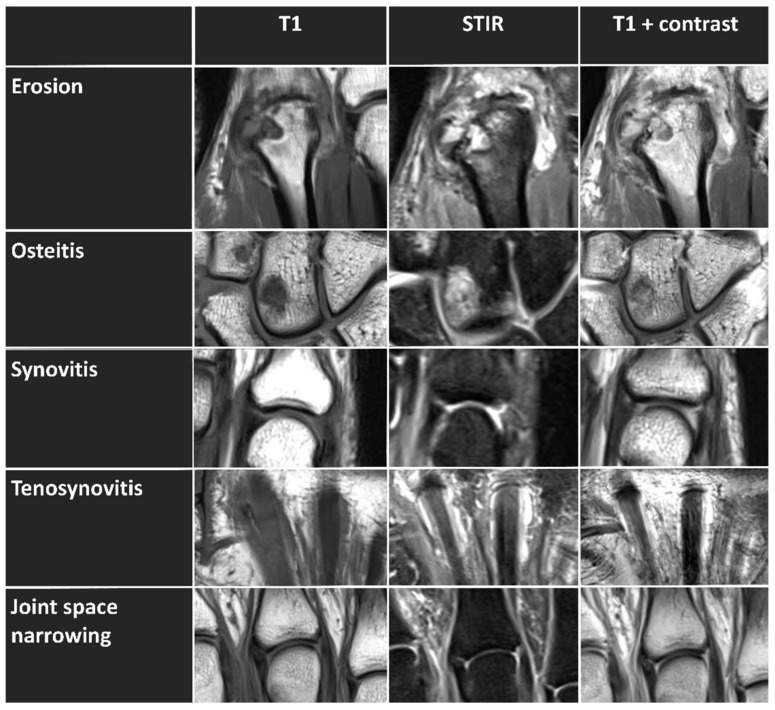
Exemplary imaging features (erosion, osteitis, synovitis, tenosynovitis, and joint space narrowing) that are scored semiquantitatively based on RAMRIS, shown in unenhanced MRT T1-weighted sequence (left column), STIR sequence (middle column), and enhanced T1-weighted postcontrast sequence (right column). Erosion is visualized at the bare area of the distal metacarpal bone of the second digit (i.e., the MCP joint base) of the right hand of a 48-year-old male patient. Osteitis is visualized in the right carpal bones with bone marrow edema in the capitate and trapezoid of a 56-year-old female patient. Synovitis is visualized in the right metacarpophalangeal joint of the fifth digit of a 53-year-old female patient. Tenosynovitis is visualized around the right flexor tendon of the second and third digit of a 75-year-old female patient. In the last row, metacarpophalangeal joints of the right hand with normal joint space without evidence of cartilage loss of a 52-year-old female patient are shown.
